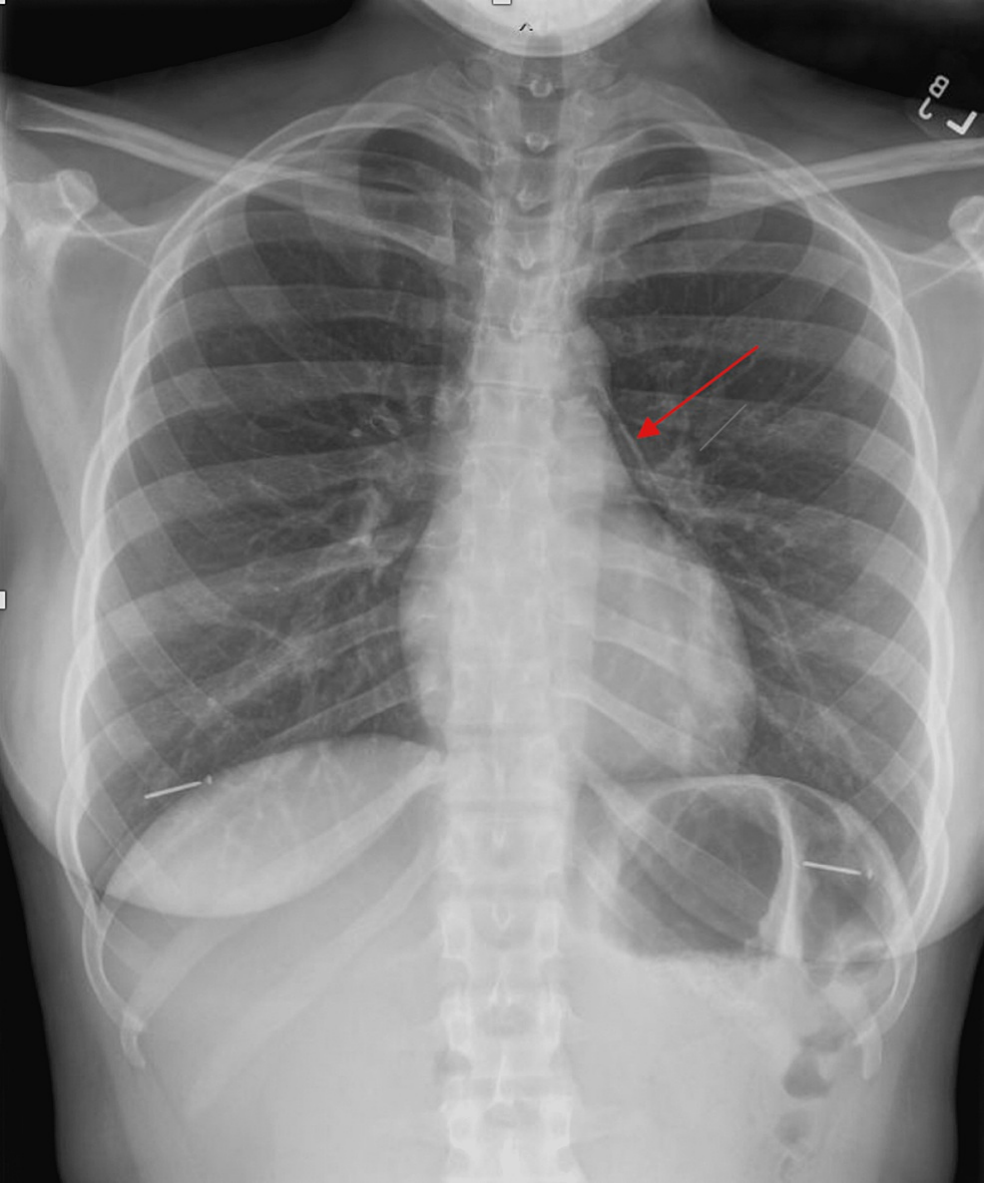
Chest X-ray showing pneumomediastinum.
